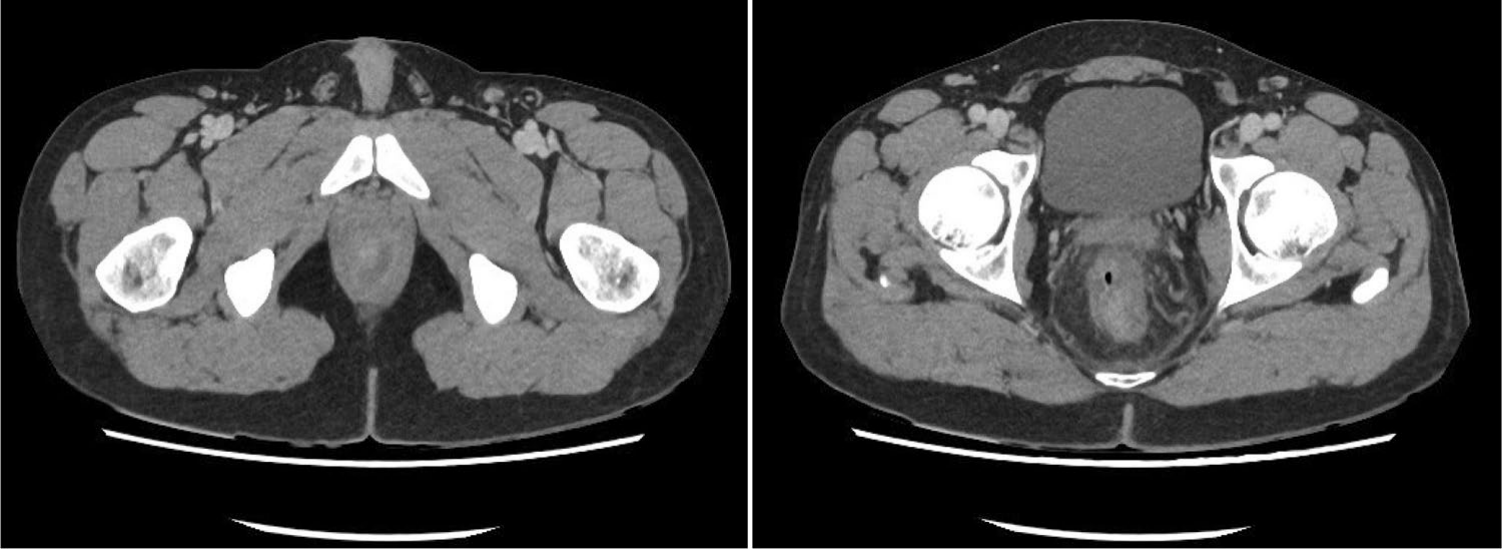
Computed tomography scan. Left lateral abscess and proctitis (patient 28)
